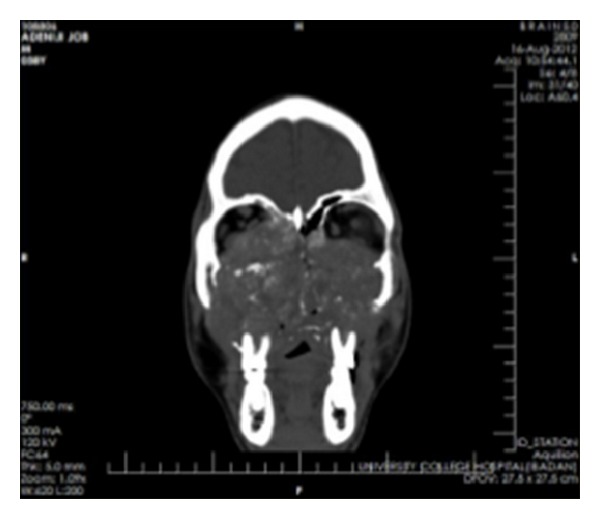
Coronal CT showing maxillary and orbital involvement.
